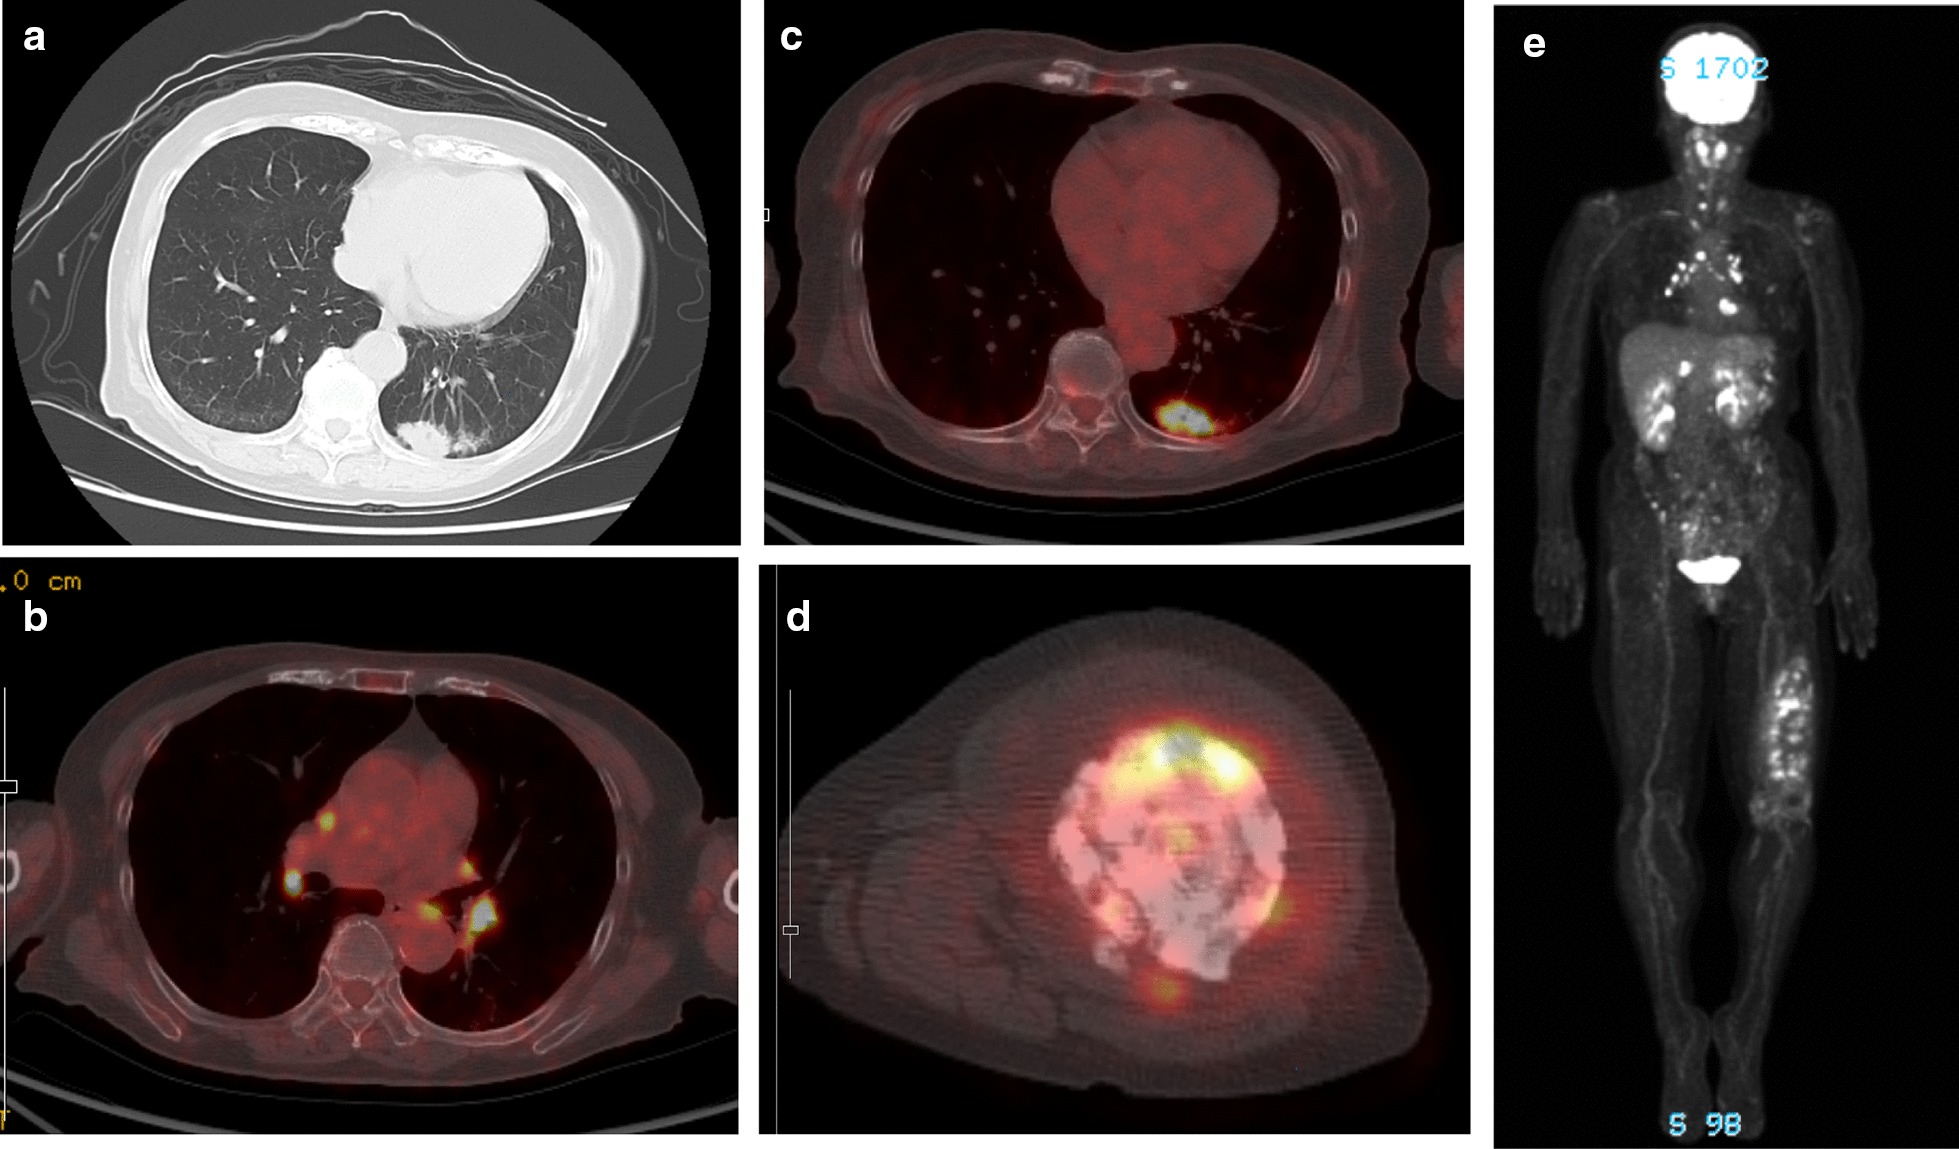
Results of Lung CT scan and 18F-FDG PET-CT scan. a Chest computed tomography scan showing a solitary pulmonary nodule in the left lower lobe. b–e 18F-FDG PET-CT scan showing simultaneous uptake in the lung, lung lymph node, cervical vertebra, thoracic vertebra, and right third rib and partially high uptake in the left distal femur
